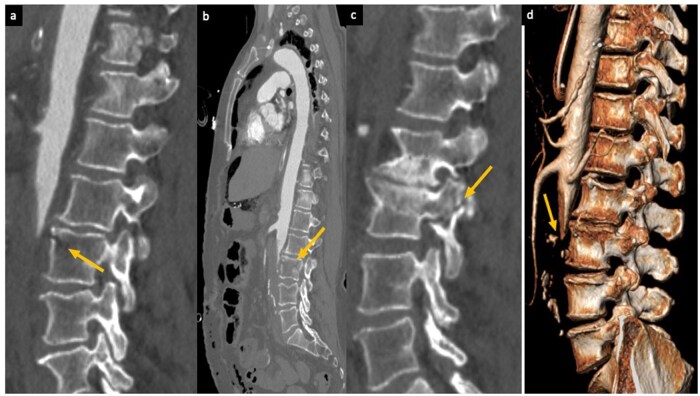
Sagittal MPR (a, b, c), and volume-rendering (d) CT images of the lumbar spine and abdominal aorta. Images in a, b, c demonstrate a horizontal fracture line at the level of L3, involving all three columns of the vertebral body (anterior, middle, and posterior), consistent with a Chance-type flexion-distraction injury. The same images show that the abrupt lack of contrast opacification in the abdominal aorta begins precisely at the level of the vertebral fracture, suggesting a traumatic origin of the vascular injury. Image (d), obtained with 3D volume rendering, clearly depicts the sudden interruption of the aortic lumen directly aligned with the fracture site at L3.
